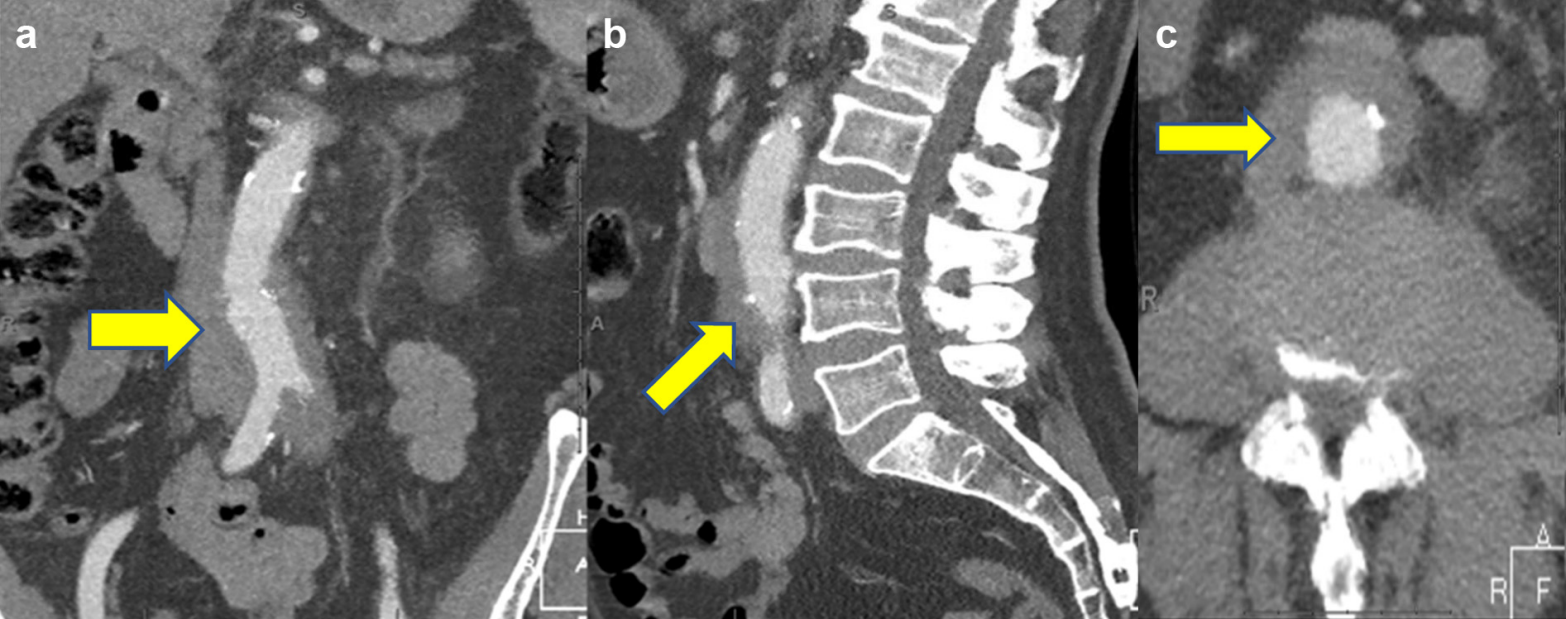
a-c, Computed tomography (CT) scans demonstrating extensive para-aortic soft tissue changes around the abdominal aorta and common iliac arteries (*yellow arrows*) causing left ureteral obstruction and moderate hydronephrosis of the left kidney, in keeping with retroperitoneal fibrosis (RPF). No associated abdominal aortic aneurysm was present.
